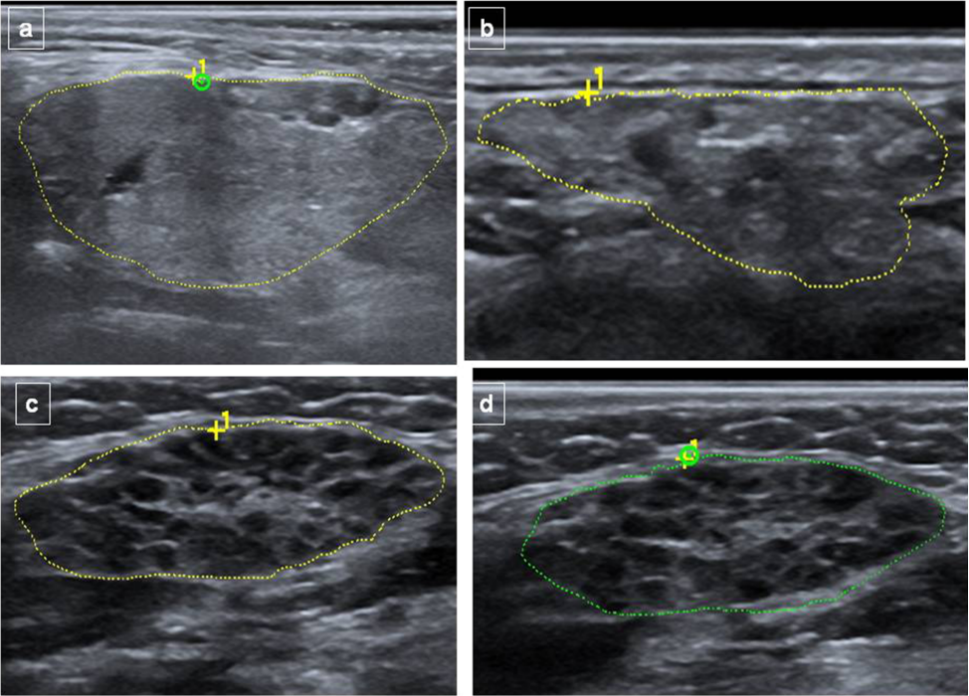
Parenchymal inhomogeneity in the submandibular glands demonstrated by ultrasonography. a Normal submandibular gland (grade 0). b Submandibular gland with evident inhomogeneity (grade 2). c, d Submandibular glands with gross inhomogeneity (grade 3)
